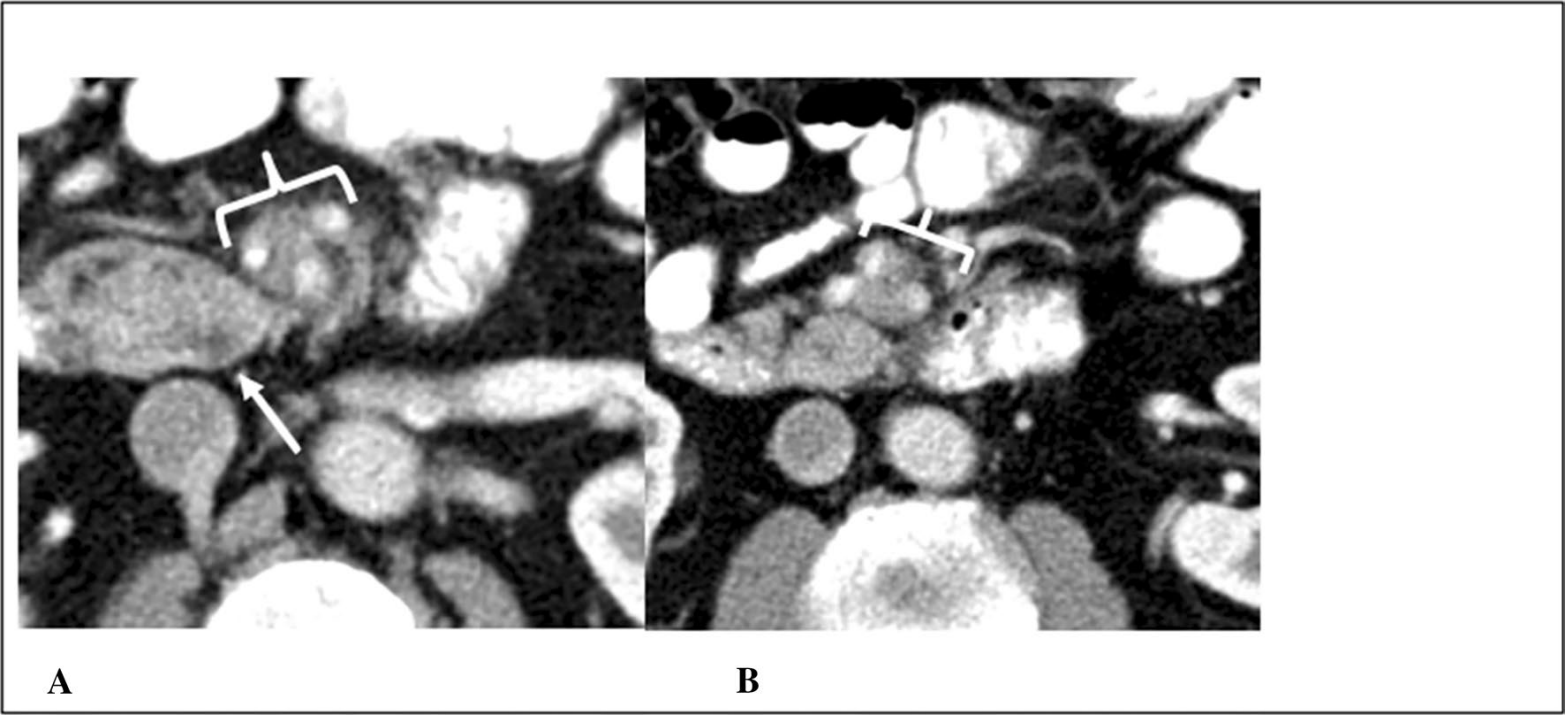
RADPEER 3 score on CT—underread and missed findings. This 69-year old male underwent imaging for evaluation of aspecific abdominal complaints. Axial postcontrast venous phase CT images at the level of the uncinate process (A) and 15 mm caudal at the root of the mesentery (B). A nearly isodense mass (arrow) is barely discernable, however, more striking is the extensive perivascular soft tissue (brackets) encasing the SMA and SMV to the level of branching in the root of the mesentery. The findings were missed on initial interpretation. Both expert readers independently reported the soft tissues encasement with high confidence. These findings must be treated as an occult mass until proven otherwise
